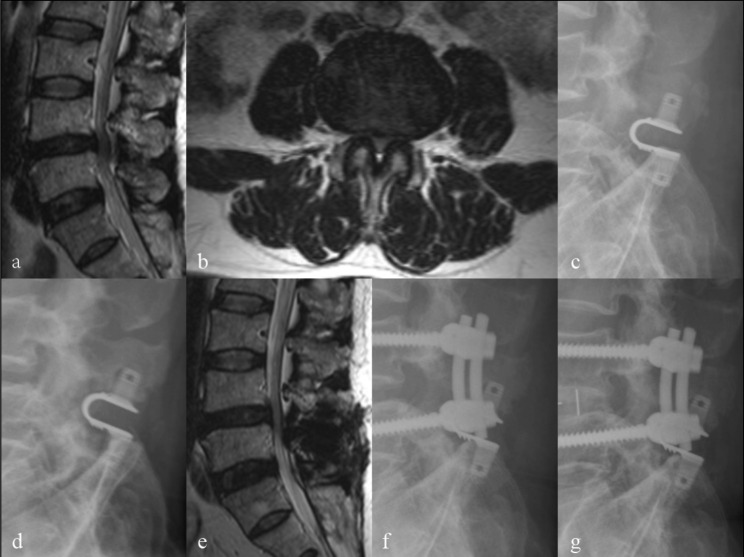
A 52-year-old patient underwent decompression at L4-5 and Coflex fixation at L4-5 for low back pain and lower extremity pain (lumbar spinal stenosis). a-b and c taken preoperative and immediately after surgery respectively. 81 months after the first operation, the patient developed numbness in left hip and lower limb. The X-ray suggested Grade 1 HO and osteolysis at the contact site of the spinous process(d). The MR imaging showed L4-L5 lumbar spinal restenosis(e). He underwent L4-5 TLIF. X ray (f) taken immediately after reoperation. X ray (g) taken 51 months after reoperation
